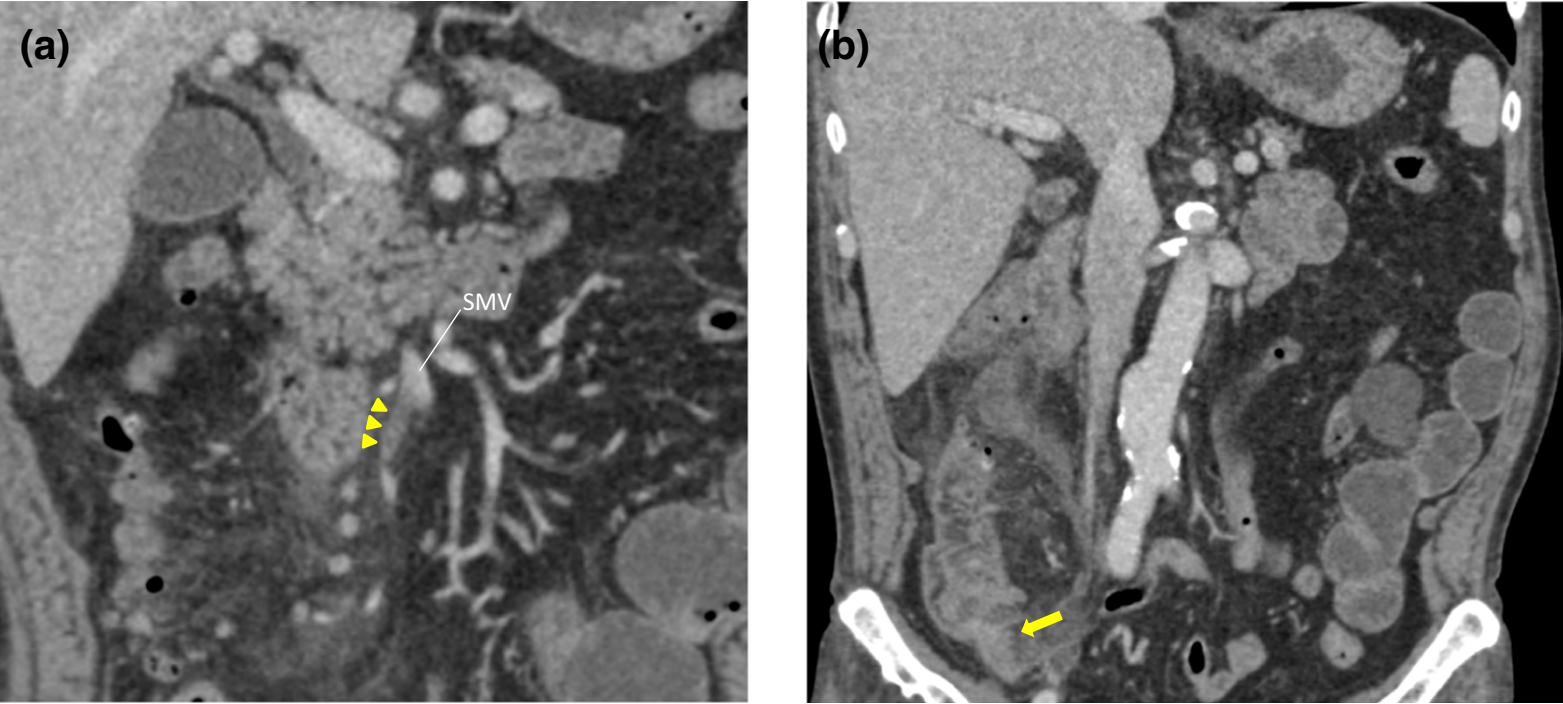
a Abdominal contrast-enhanced CT on admission. Thrombus is found from the ileocolic vein to the superior mesenteric vein. Arrowheads: mesenteric vein thrombus. b Abdominal contrast-enhanced CT on admission. Periappendiceal fat standings and unevenness of the appendix wall are found. Appendiceal diverticulitis is suspected. Arrow: unevenness of the appendix wall
